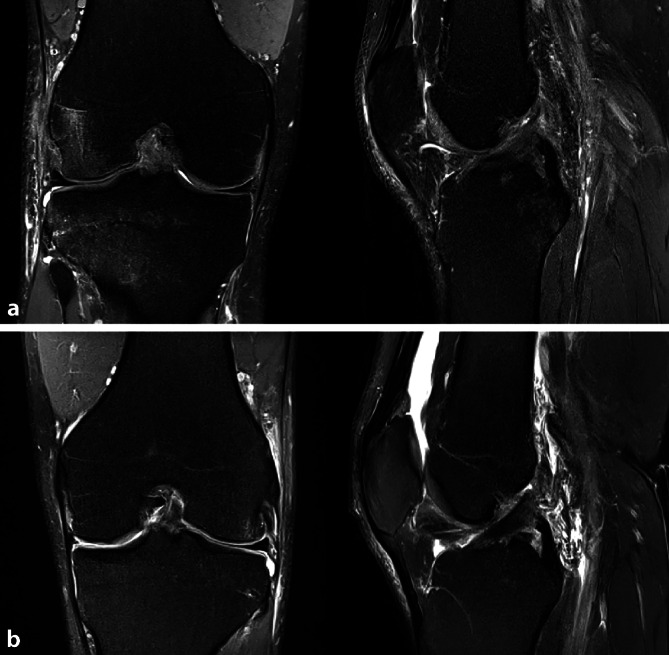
### Computertomographie (CT)
